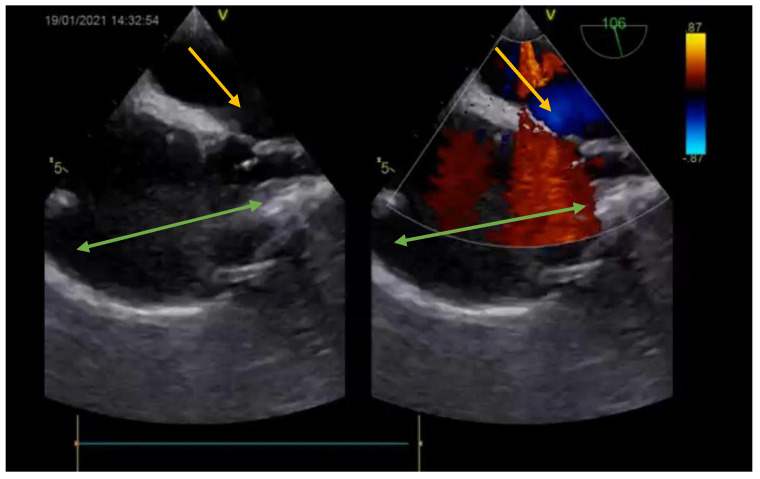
Postoperative echocardiography (bicaval view—Doppler) showing the closed ASD (no leaks—yellow arrow) and the enlarged SVC and right atrium (green arrow).
